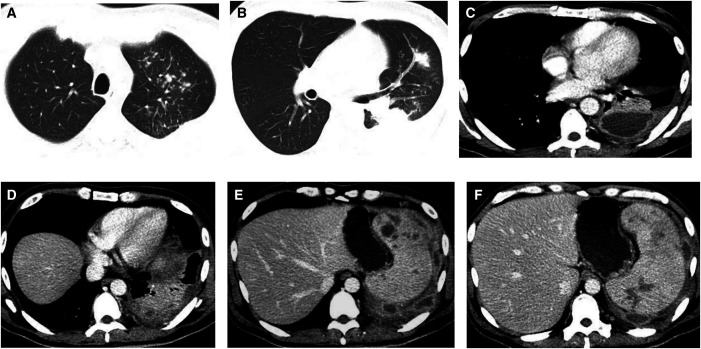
The thoracic and abdominal computed tomography of 2 months before admission. (A–D) Exacerbated lung lesions, which predominantly manifested as nodular changes in the left upper lobe, along with persistent left pleural effusion. (E, F) The spleen was enlarged and contained multiple irregular, low-density areas with heterogeneous enhancement.
